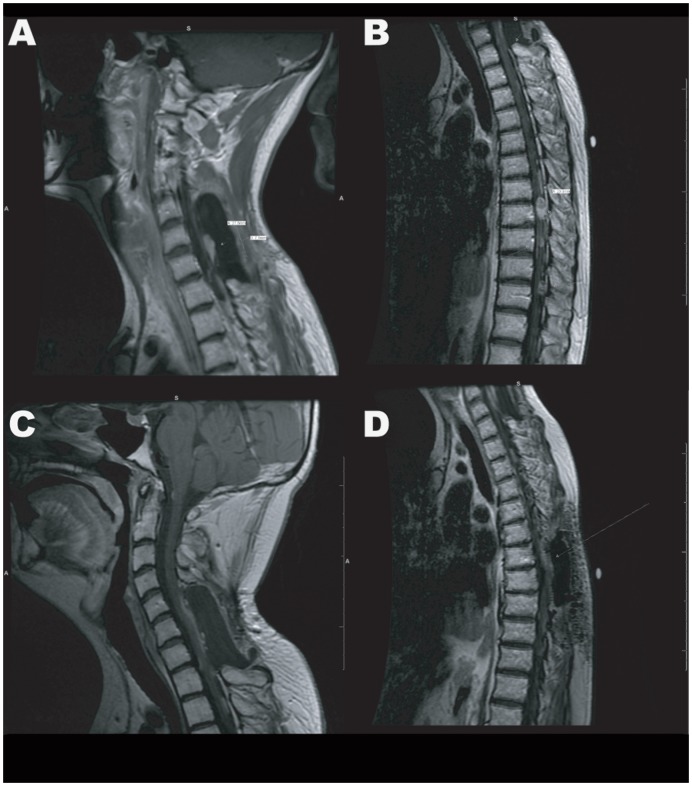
MRI Images of Cervical and Thoracic Spine. A) 2009 MRI of the cervical spine showing recurrence in the surgical area. B) 2009 MRI of the thoracic spine showing progression of the main lesion measuring 23.9 mm, and the appearance of several other smaller lesions. C and D) 2010 MRI of the cervical and thoracic spine showing tumor regression following a treatment with irinotecan and bevacizumab.
